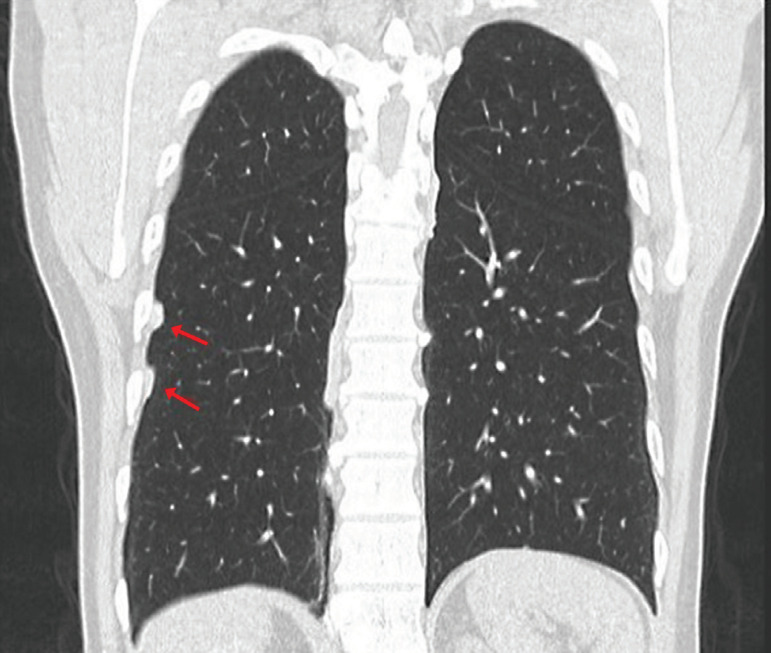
Coronal reconstruction of chest computed tomography showing at least two pleural plaques with inner calcifications on the anterolateral wall of the right hemithorax.
